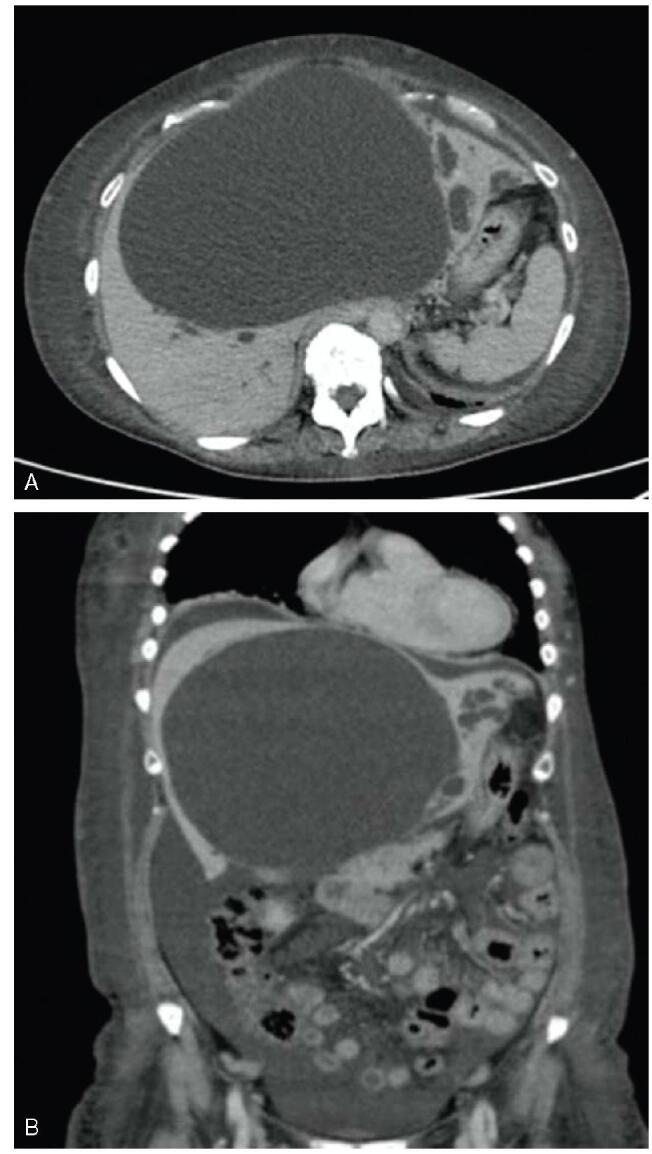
Figure 1. Abdominal contrast-enhanced computed tomography scans obtained during hospitalization. A) A large cyst is visible in the liver. Dilation of the intrahepatic bile ducts are also observed. B) Presence of significant ascites.
